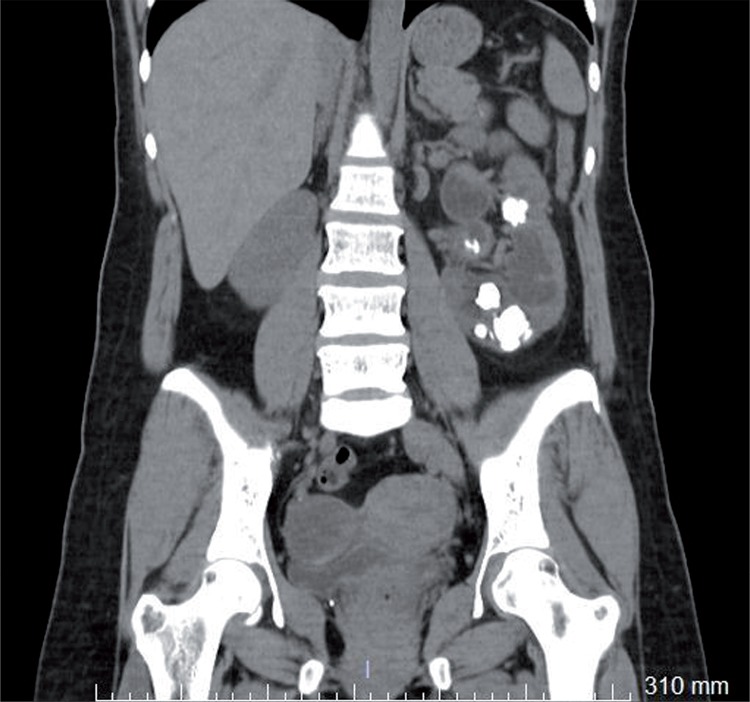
Computed tomography scan with multiple calculus in left kidney.
